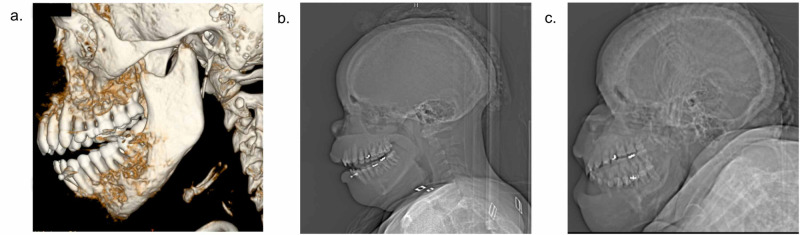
CT face and head demonstrate the patient's distorted anatomy and disease progression CT face (a) and head (b) with reconstruction when the patient was at the age of 28 demonstrated diffuse enlargement of bilateral mandibles, maxillas, hard palate, orbital walls, and bony thickening of the calvarium and skull base, with superimposed bony permeative change and periosteal reaction. The decreasing size of bilateral maxillary sinuses was also noted, secondary to bony overgrowth of the maxilla. (c) current patient facial features at age 37 with recent CT head showing diffuse osseous expansion, heterogeneous mineralization, and multifocal rounded foci of sclerosis and lucency, most prominent in the frontal calvarium and facial bone CT: computed tomography
